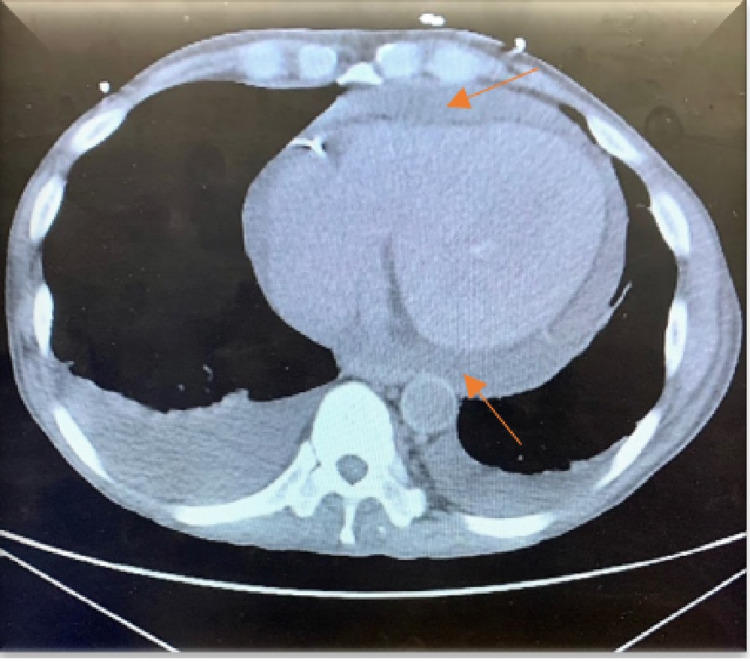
CT chest soft tissue window showing pericardial effusion.
